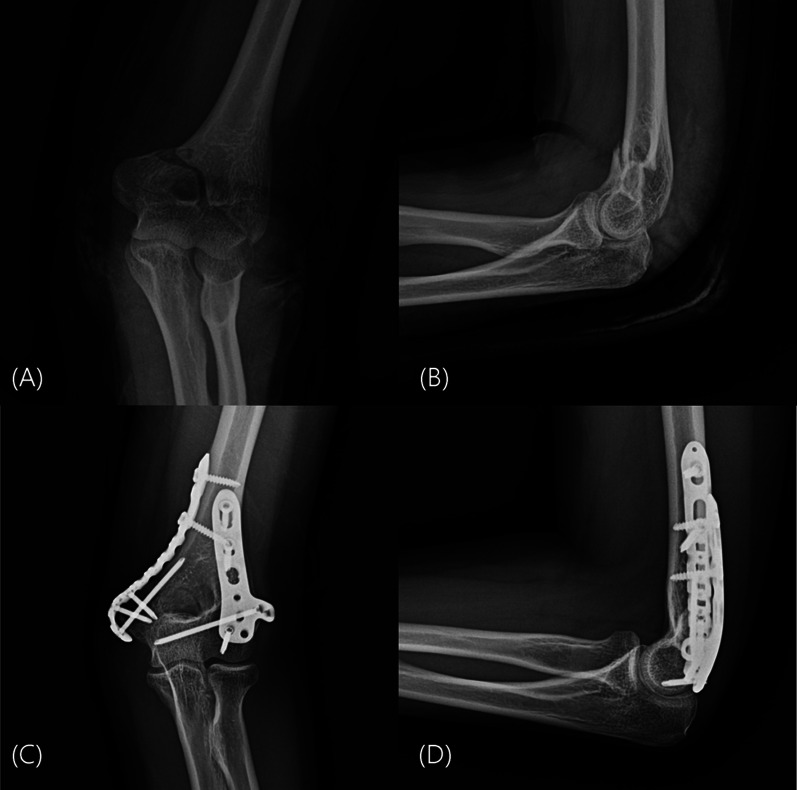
A 37-year-old female patient treated with double-plate fixation. A, B Preoperative radiographs showed an extra-articular distal humerus fracture. C, D Postoperative radiographs obtained at final follow-up demonstrated bony union
